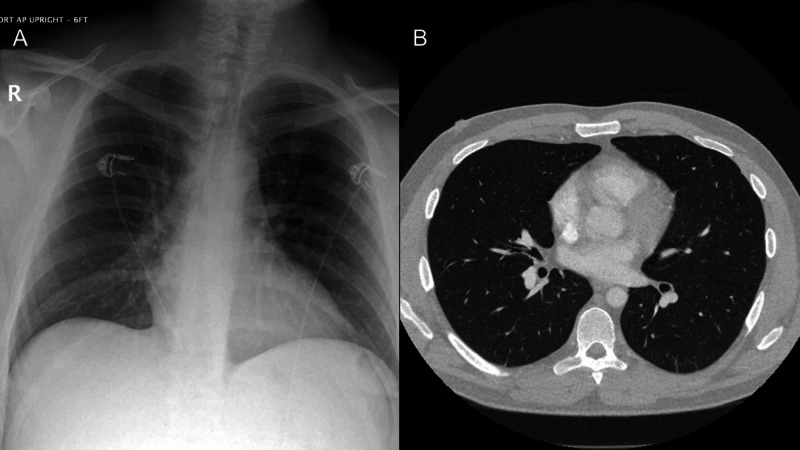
(A) Chest X-ray and (B) chest CT are both negative for any findings of interstitial or lobar pneumonia
